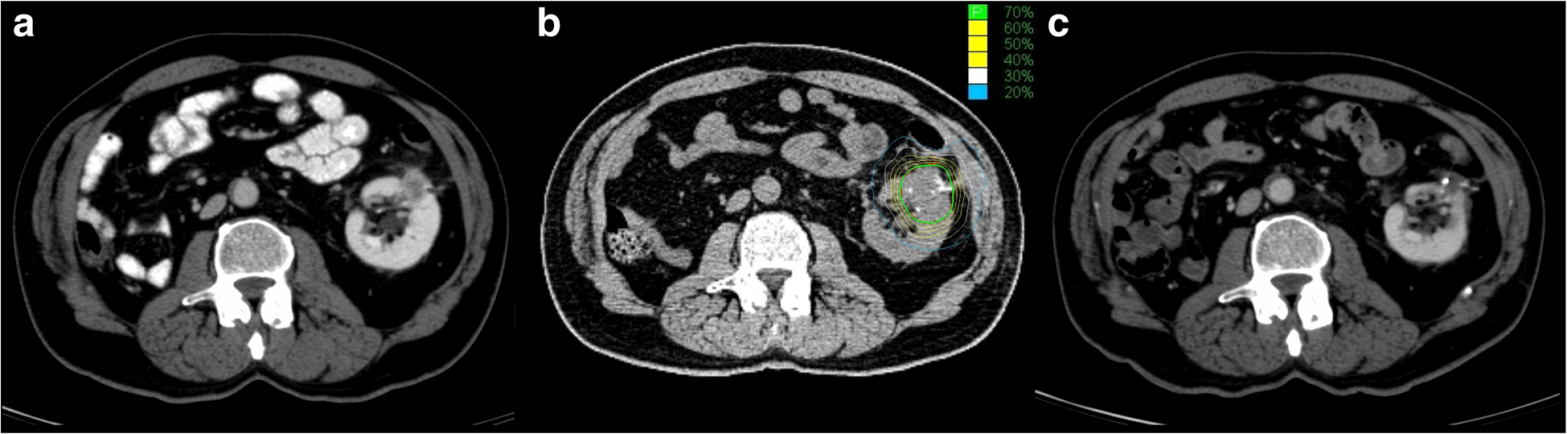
A representative case of a renal cell carcinoma. a Demonstrates a representative image before radiotherapy and b the treatment plan with exemplary planning computed tomography image in axial view with the treatment plan. Single fraction 25 Gy was prescribed to 70% isodose line (in green) to treat planning target volume shown in red line. The yellow and white circle lines represent the remaining isodose lines until 20% in blue. c shows an image 2 years after robotic stereotactic ablative radiotherapy
